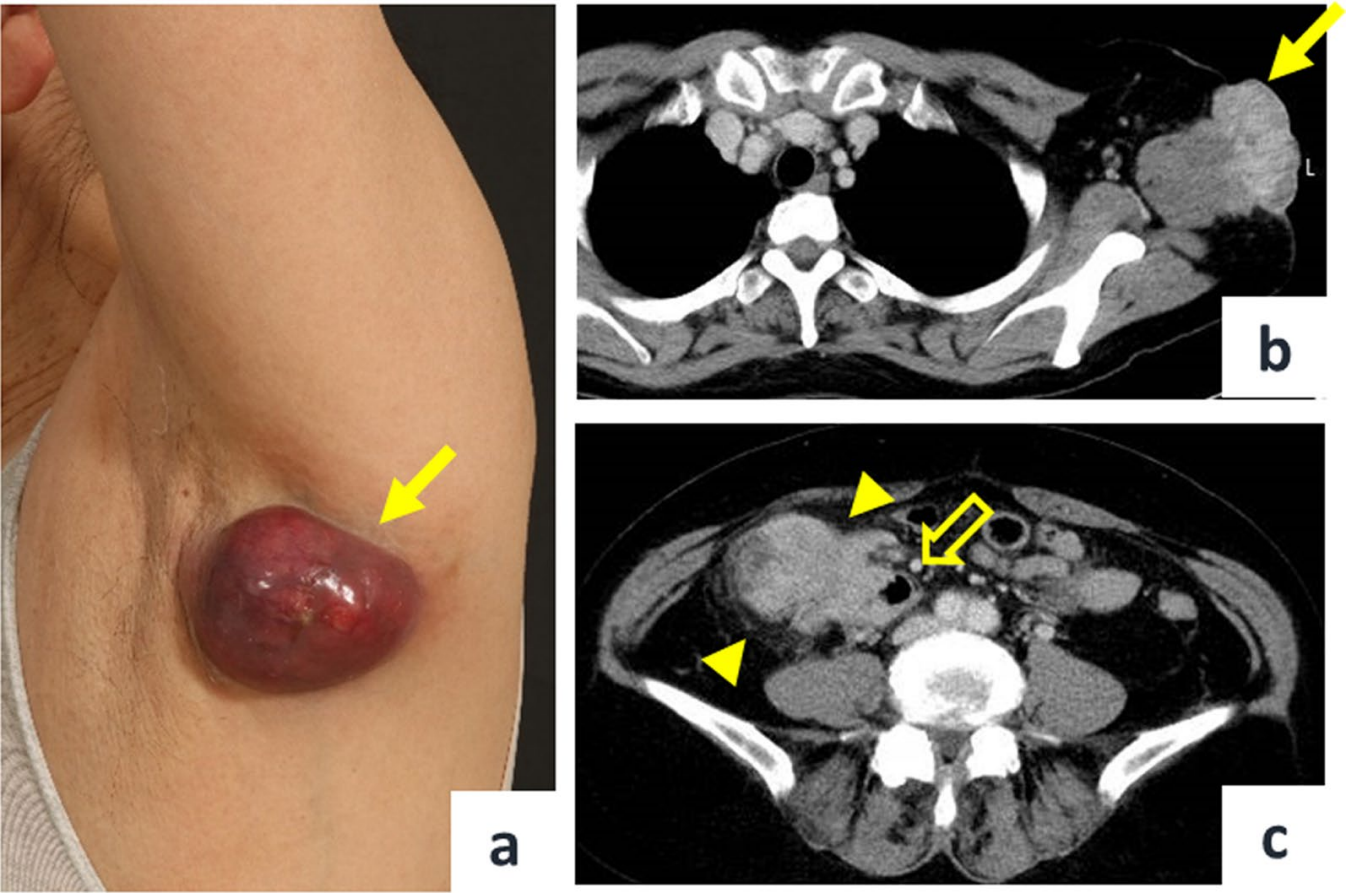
Pre-treatment clinical findings. a Cutaneous tumor measuring 5 cm in diameter located on the left axillary skin (solid arrow). b CT scan of the chest shows the cutaneous tumor in the left axilla with the invasion of the left teres major muscle (solid arrow). c CT scan of the abdomen and pelvis shows the circumferential enhancing wall thickening of the ascending colon (arrowheads) with invasion of the duodenum (open arrow)
